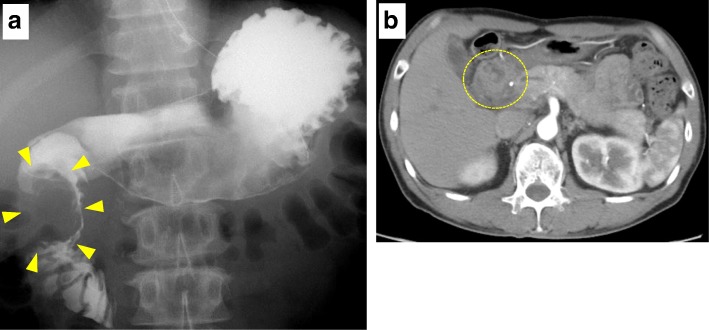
a Upper gastrointestinal series demonstrated a filling defect in the duodenum, while the inside of the cystic lesion was not contrasted. b Follow-up CT on the 27th day after TAE showed that the cyst decreased in size
